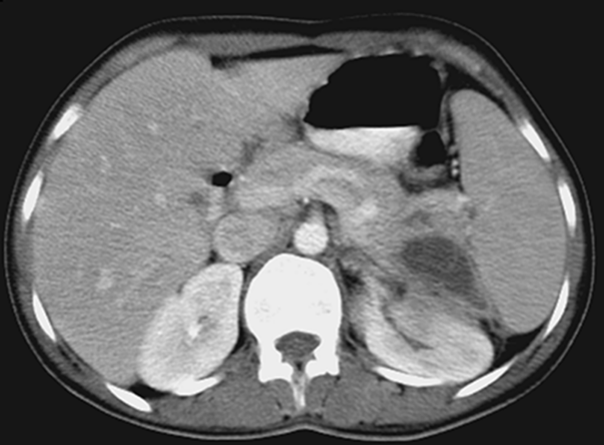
Contrast-enhanced computed tomography scan of the abdomen showing cystic lesions with a curvilinear laminated membrane at the tail of the pancreas.
